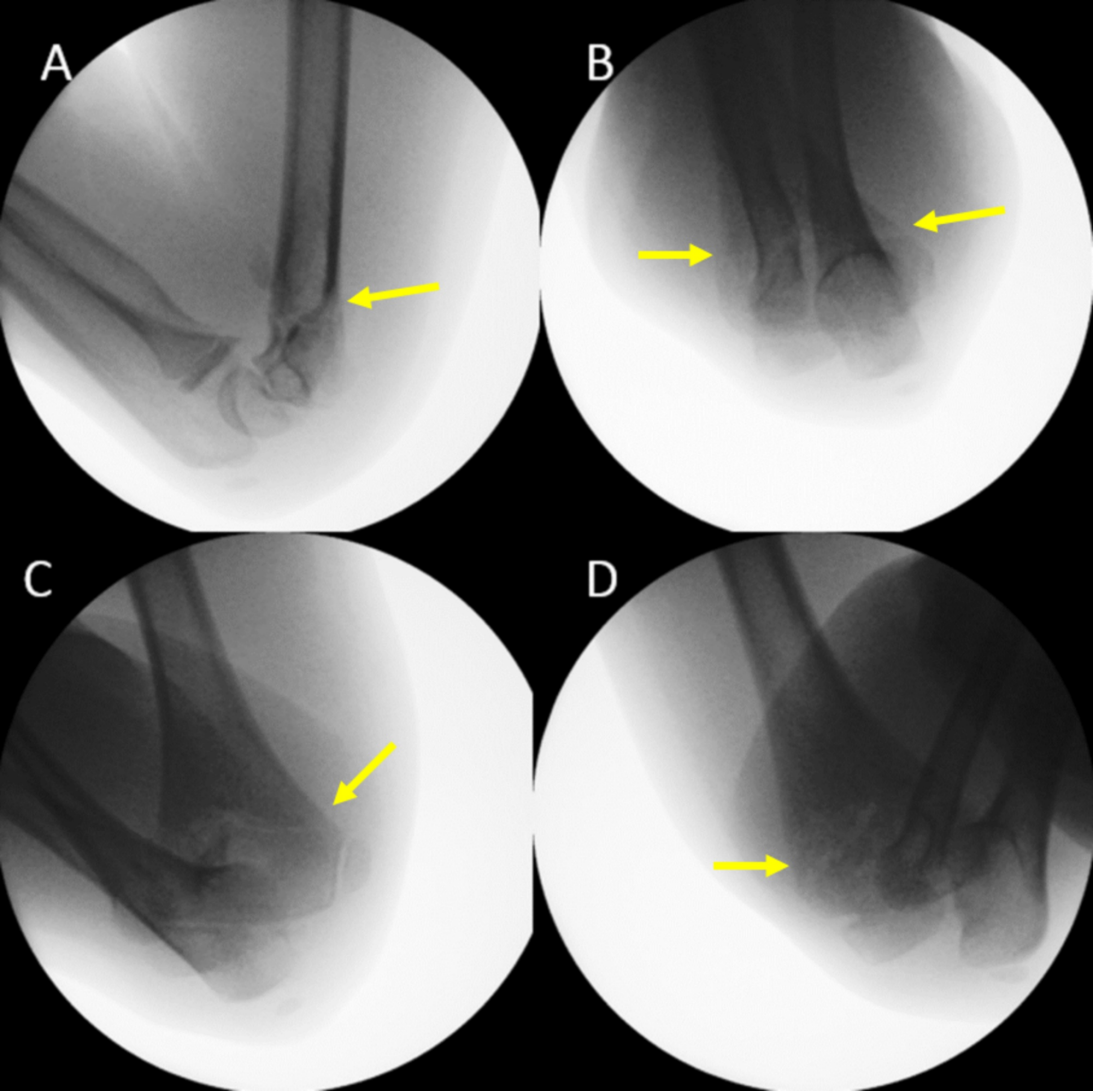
Radiographic controll of fracture reduction under general anesthesia Lateral view (A), Jones view (B), external view (C), internal oblique view (D) of the elbow. Only a continuous diaphyseal-metaphyseal contour of the humerus on all projections is accepted. Arrows indicate the location of the fracture after reduction.
